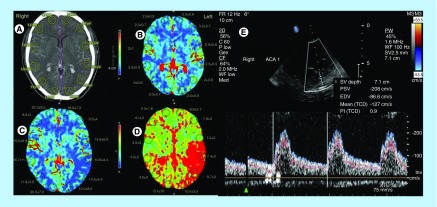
A 41-year-old male patient (S4 patient in text) with subarachnoid hemorrhage caused by rupture of anterior communicating artery aneurysm, developed vasospasm and 8 days after the bleeding had small area with ischemic lesion to the right frontal. (A) Computed tomography scan in the second day after rupture, showed aneurismal subarachnoid hemorrhage without ischemia, computed tomography perfusion in the same time. (B) Blood volume map; (C) cerebral blood flow map and (D) time to peak map images in the same patient showed normal brain parenchyma and regional cerebral blood volume, but abnormal regional cerebral blood flow and mean transit time, suggesting an area of low perfusion; (E) transcranial Doppler in the right anterior cerebral artery; pulsatility index was normal (PI = 0.9).
